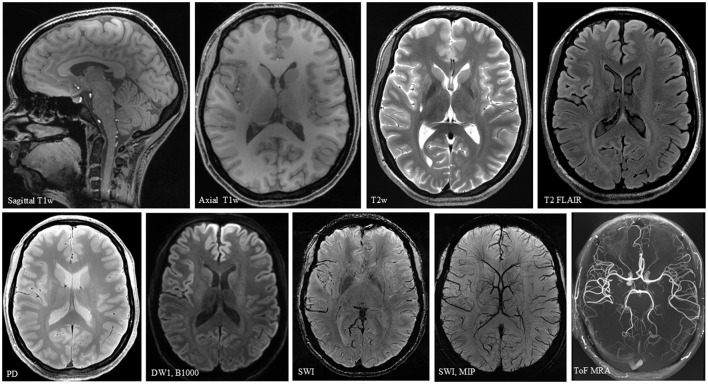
Images of the brain obtained from a subject scanned with the 8Tx64Rx (pTx). These include a sagittal T1-weighted acquisition through the midline structures; 3D T_1_-weighted, T_2_ TSE, T_2_ FLAIR, PD, and DWI (*b* = 1,000 s/mm^2^), SWI and SWI MIP axial acquisitions acquired at a plane through the level of basal ganglia, insula and corona radiata; and TOF MRA (MIP) through the Circle of Willis.
